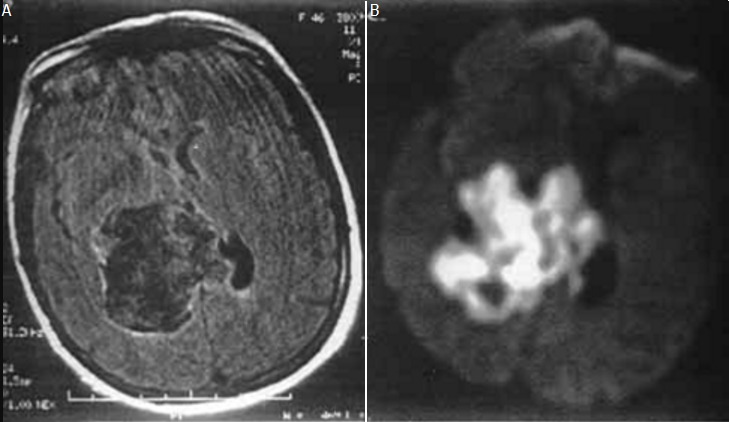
Aspect du kyste épidermoïde intraventriculaire: (A) en IRM (séquences FLAIR; (B) diffusion. La séquence FLAIR permet d’affirmer la nature « tissulaire » du kyste. Le kyste est hyperintense en séquence de diffusion. Ceci permet de clairement différencier le kyste épidermoïde du kyste arachnoïdien
